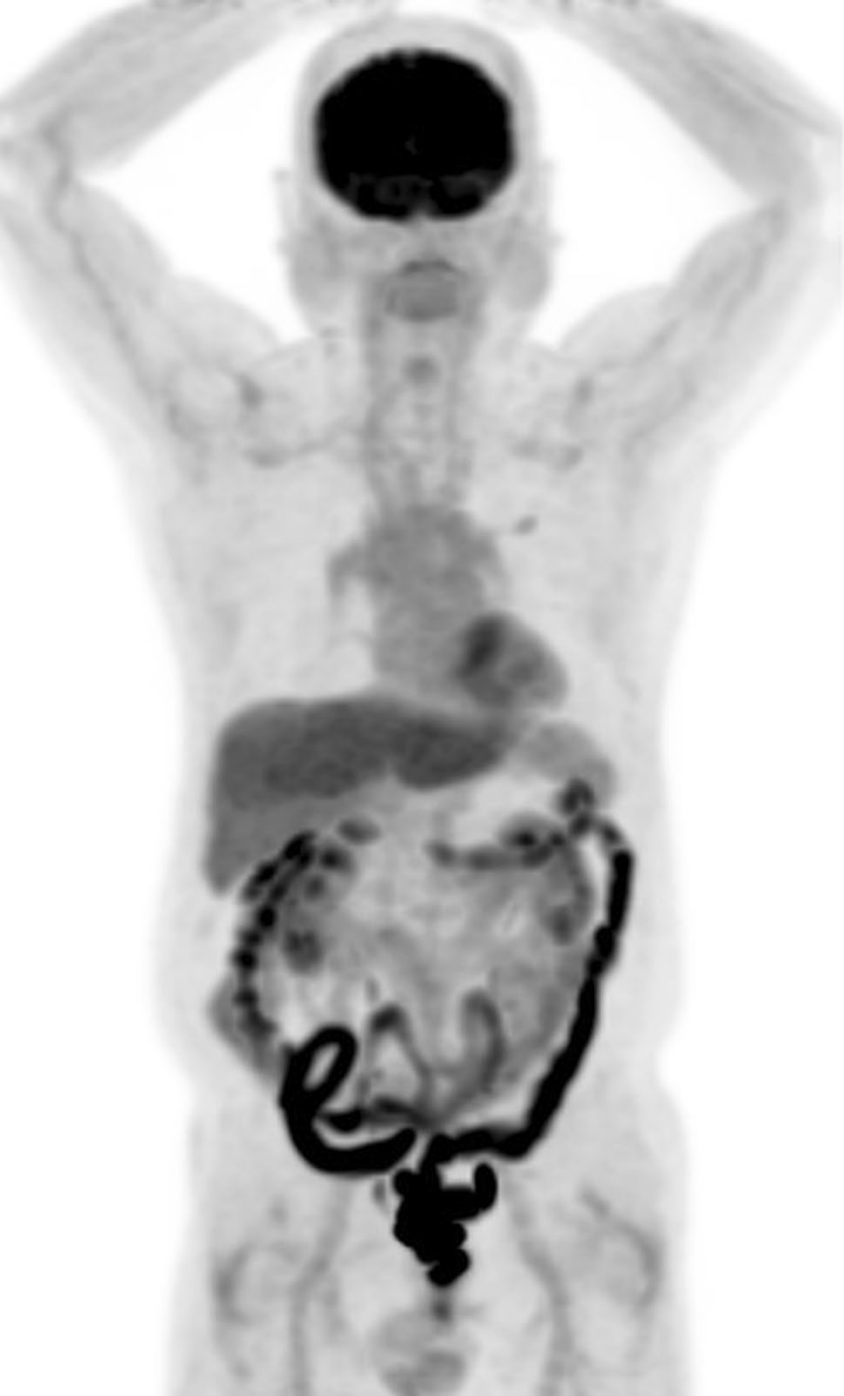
Increased bowel FDG distribution in a patient using metformin
